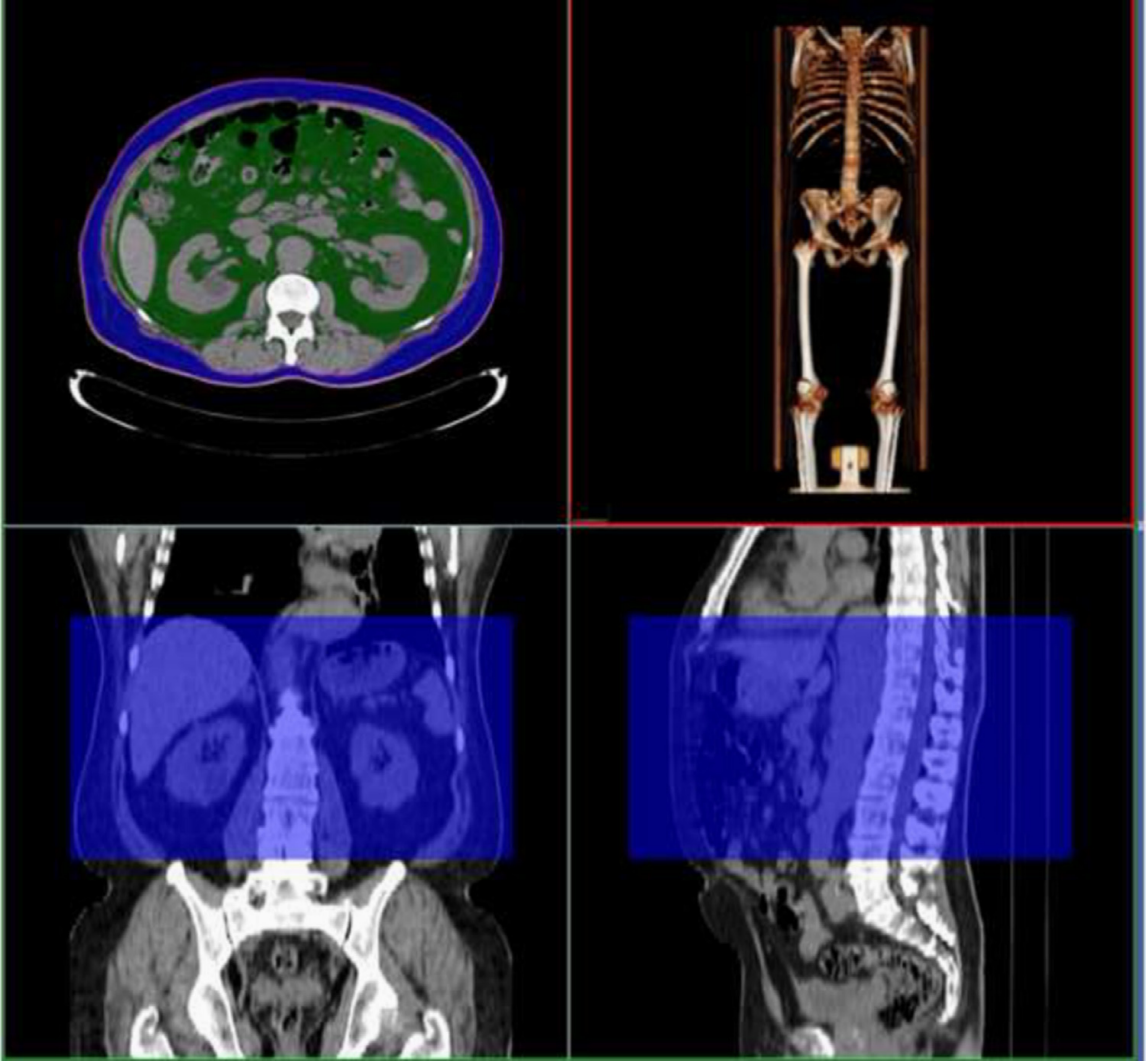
These abdominal CT images are examples of the visceral adipose tissue volume measurement. VATV is highlighted green in the upper left image.
